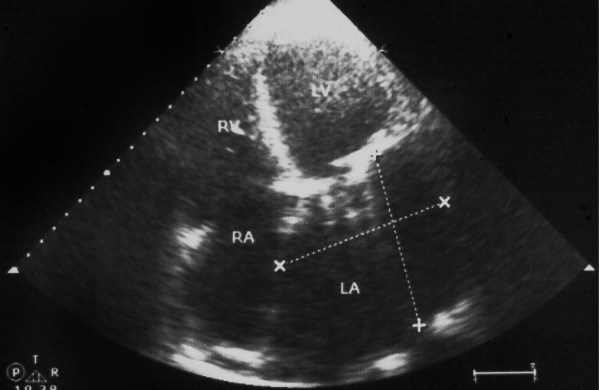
Postoperative echocardiography showing a reduction of left atrial size.
